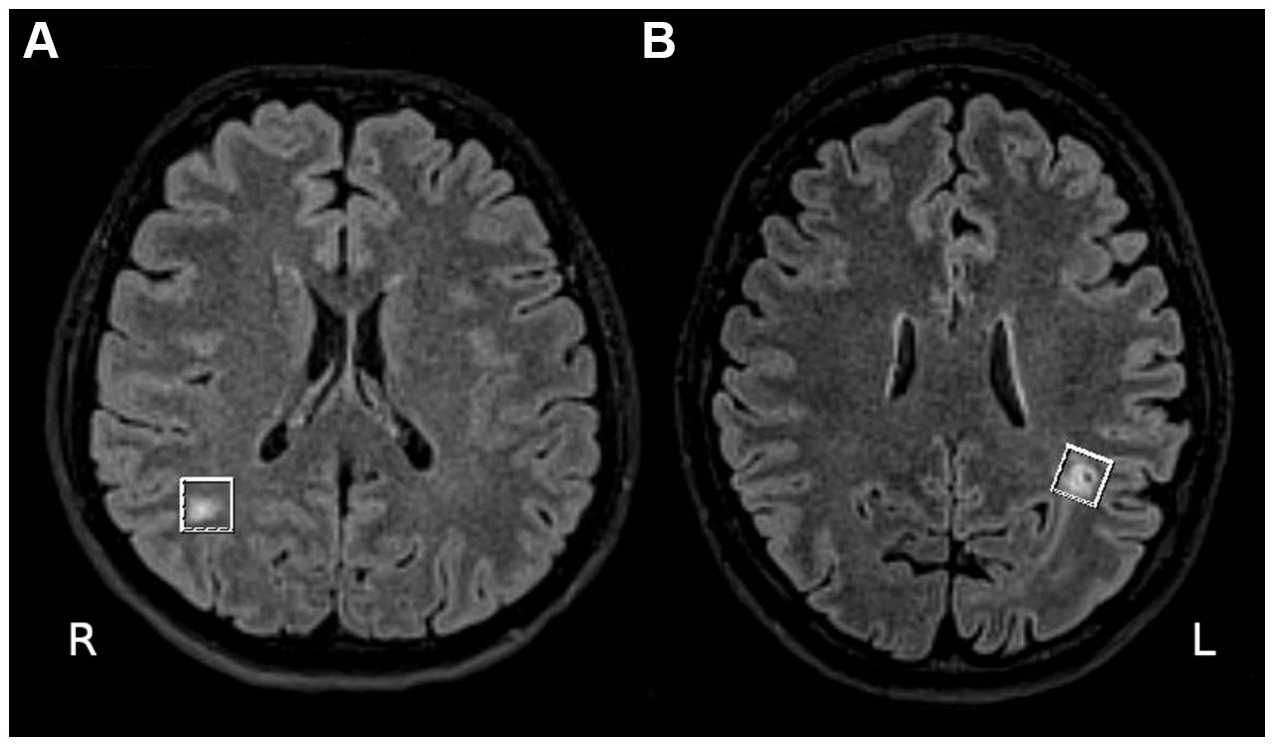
(A) A 47-year-old woman with an aura free migraine. The axial fluid-attenuated inversion recovery (FLAIR) MR image shows a large white matter lesion (WML) in the right occipital lobe with normal appearing white matter (NAWM) on the contralateral side. (B) A 30-year-old female patient with relapsing–remitting multiple sclerosis. The axial FLAIR MR image shows a large WML in the left supraventricular parietal lobe with NAWM on the contralateral side.
